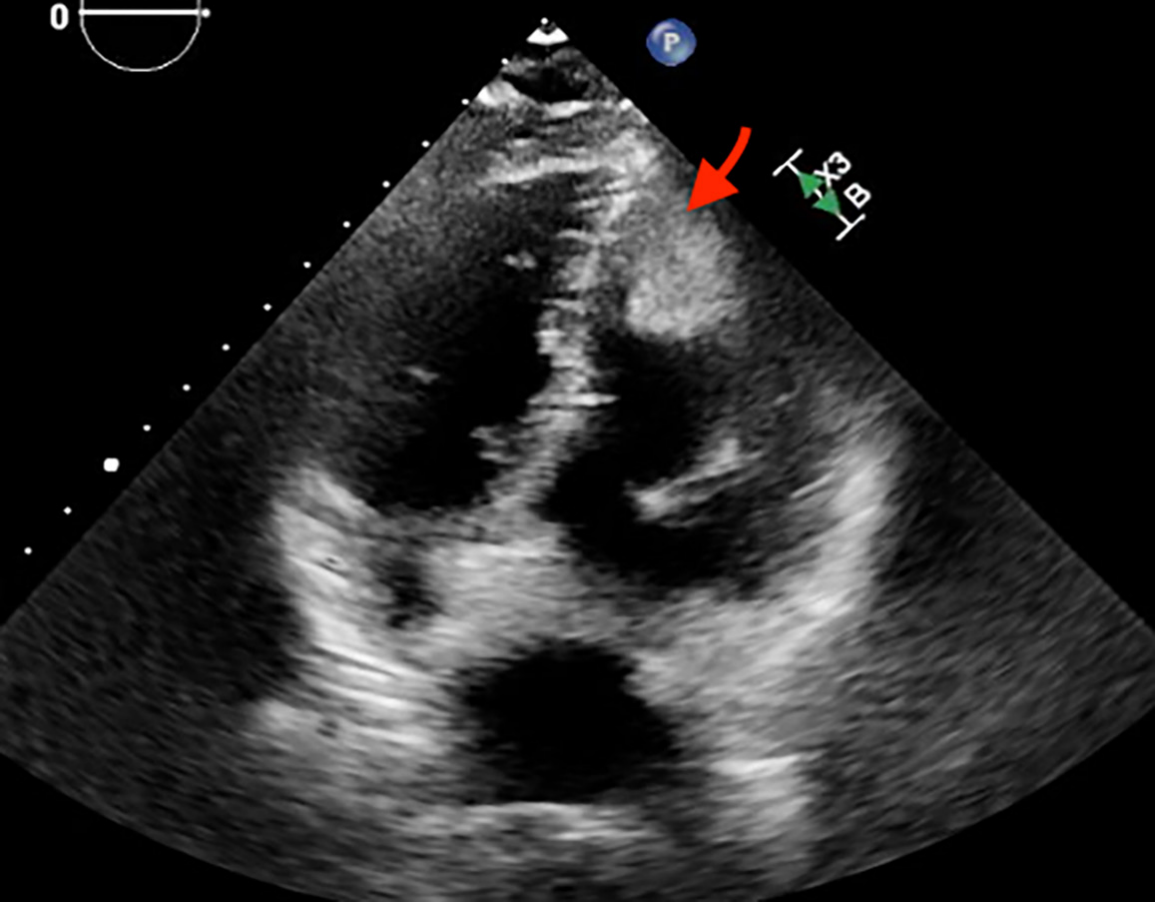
Transthoracic echocardiogram four chamber view demonstrating an irregular distal left ventricular mass (arrow).
